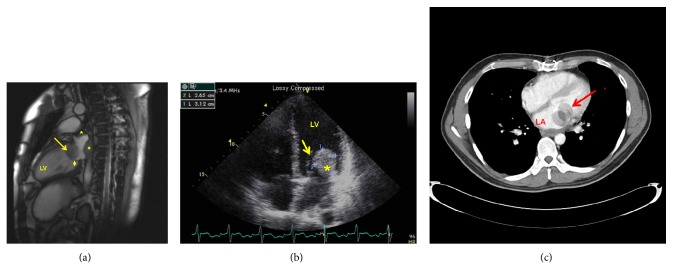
Presentation 2. (a) MRI showing mass in the left side of the heart pressing on the mitral valve (arrow head) and two other masses in the left atrium indicated (asterisks). (b) TEE showing a mobile, echogenic mass (asterisk) in the left heart prolapsing through the mitral valve (arrow) into the ventricle. (c) Nongated CT-scan captured the mass (arrow) in motion in the left side of the heart. LA: left atrium. LV: left ventricle.
